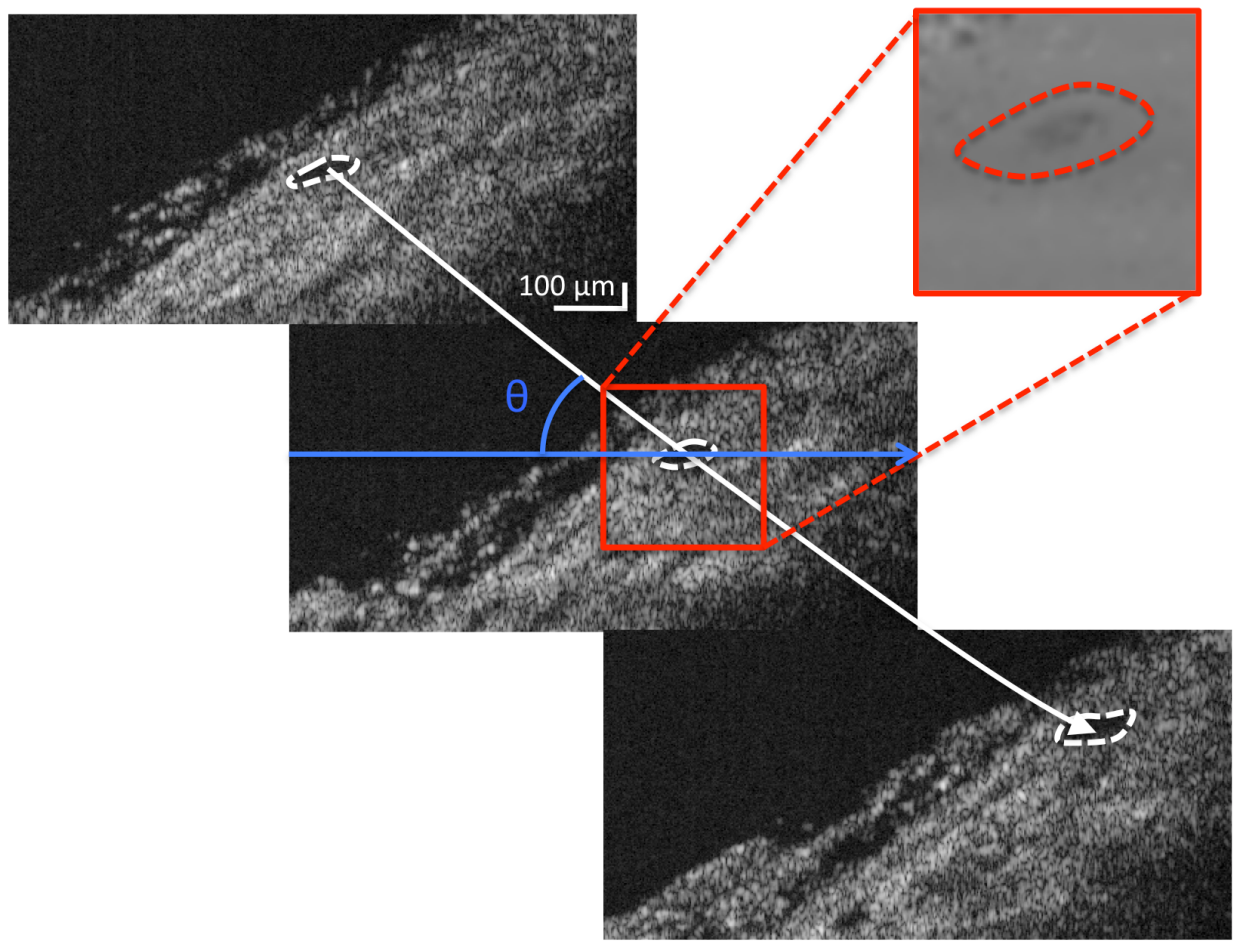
Determining volumetric flow. Adjacent B-scan images containing the vessels of interest are identified and marked (dashed white line) to determine the centroid. A line (white line) was fitted through the centroid to determine the angle of the vessel, θ, with respect to the OCT imaging beam (blue arrow). This allows us to integrate the flow within the Doppler information (red) and correct for the angle of flow with respect to the OCT scanning beam.
